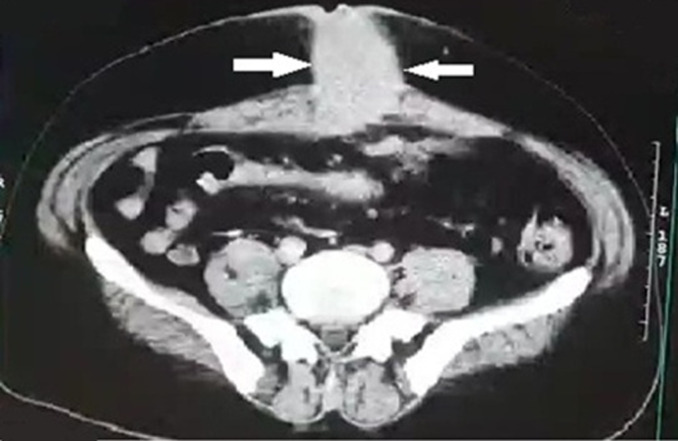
CT-scan of the abdomen; this image shows an umbilical mass
